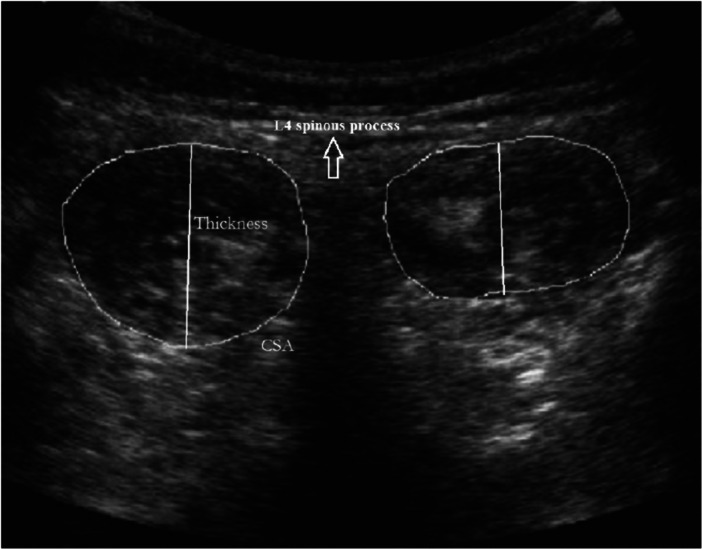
Bilateral ultrasound imaging of the lumbar multifidus muscle at the L4 vertebral level during measurement.
